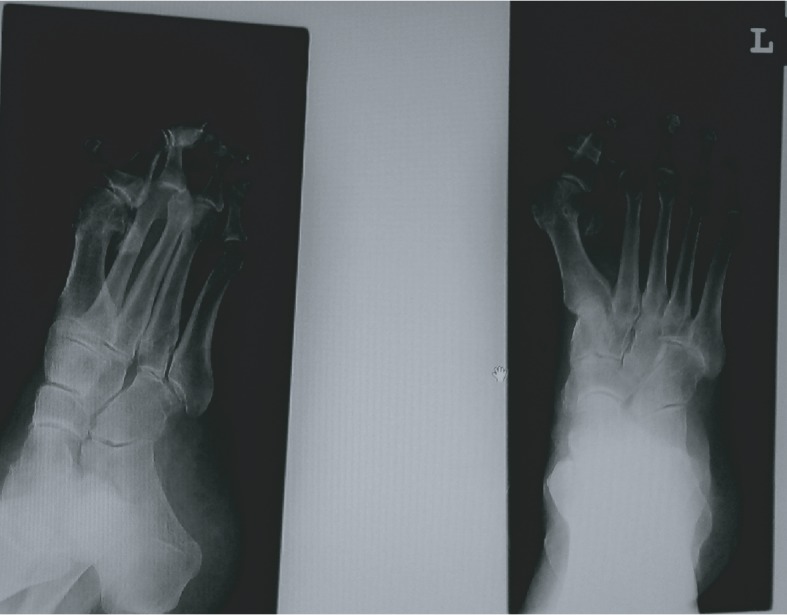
X-ray of the patient's left foot before the operation.
